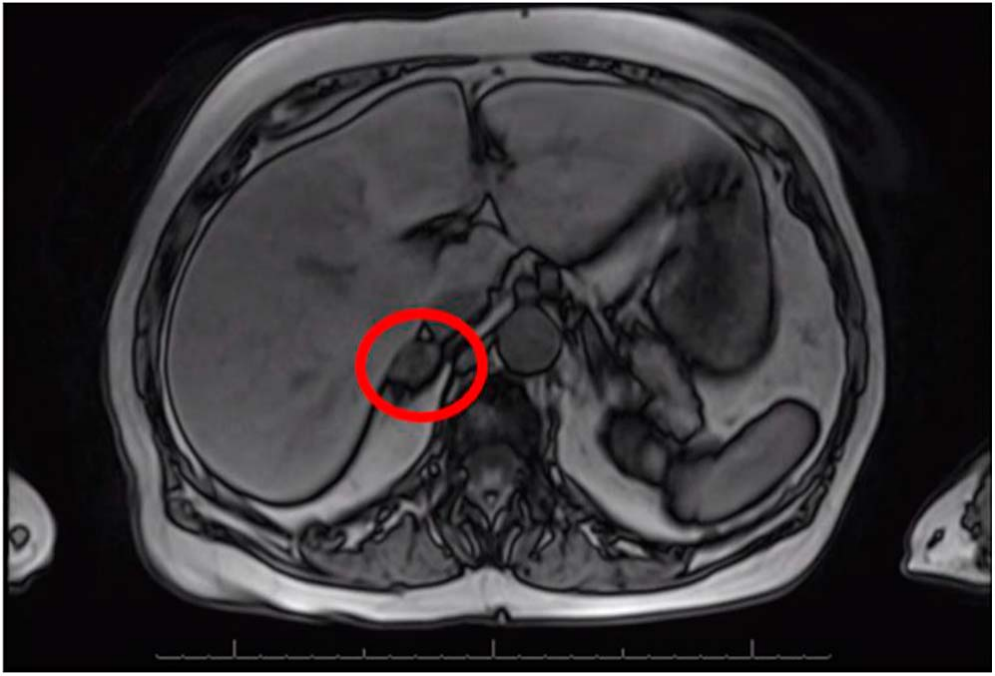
MRI abdomen. Axial T1, post-contrast and fat saturation.
